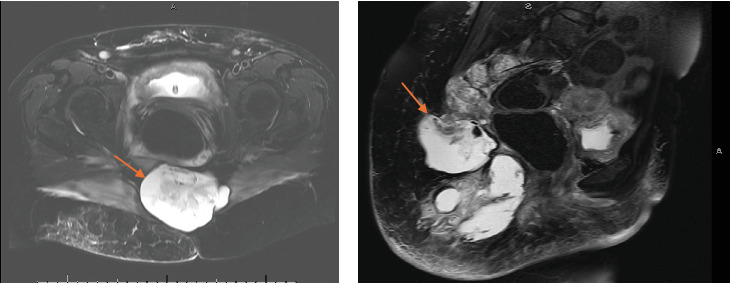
The 6-week postoperative MRI showed a solid cystic mass (arrows) infiltrating the sacrum with solid cranial and cystic caudal components. (a) Sagittal view. (b) Coronal view.
